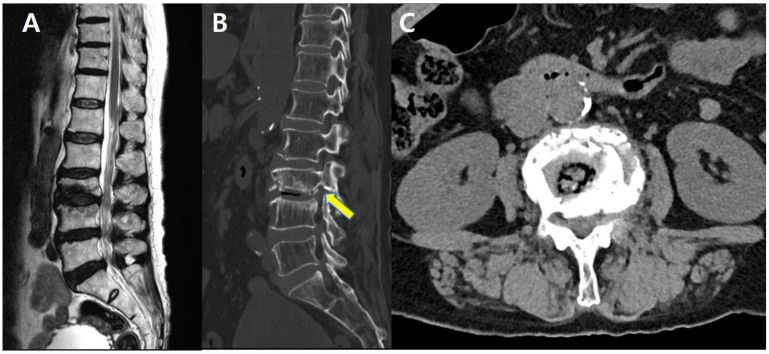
An 82-year-old female patient complains of severe radiating pain in the left leg due to an L3 osteoporotic compression fracture. (A) MRI shows a fracture of the L3 inferior end plate. (B) At the beak point, the fractured bone is obstructing the foramen (yellow arrow). (C) CT axial images also reveal the fracture.
